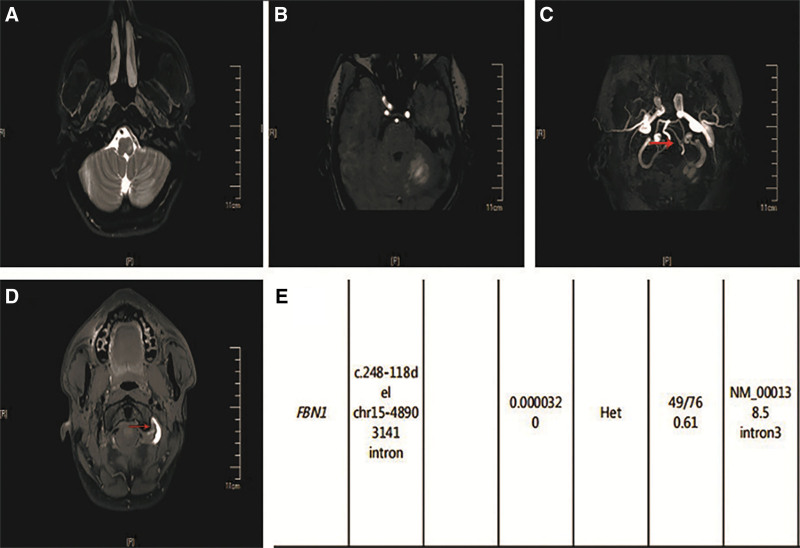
Radiologic findings and gene tests of the patient. (A, B) Hyperintense signals on MRI were detected in the brainstem and left cerebellar hemispheres. (C) High-resolution vessel wall imaging (HRVW) MRI showed fenestration of the V4 segment of the left vertebral artery. (D) HRVW MRI showed vertebral artery dissection of the V3 segment with possible intramural hematoma. (E) Results of NGS for the patient. The patient had a heterozygous mutation on chr15-48903141. MRI = magnetic resonance imaging, NGS = next-generation sequencing.
